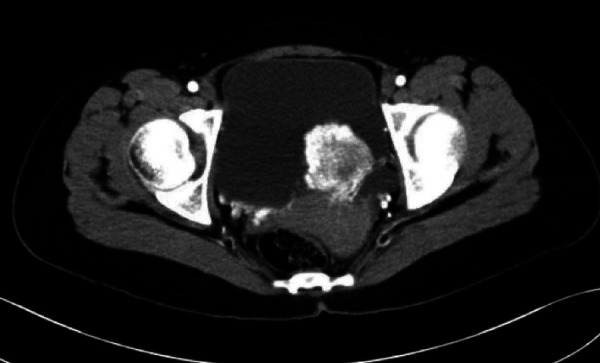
The arterial phase enhanced CT scan of the patient whose PUB tumor was located in left posterior wall.
